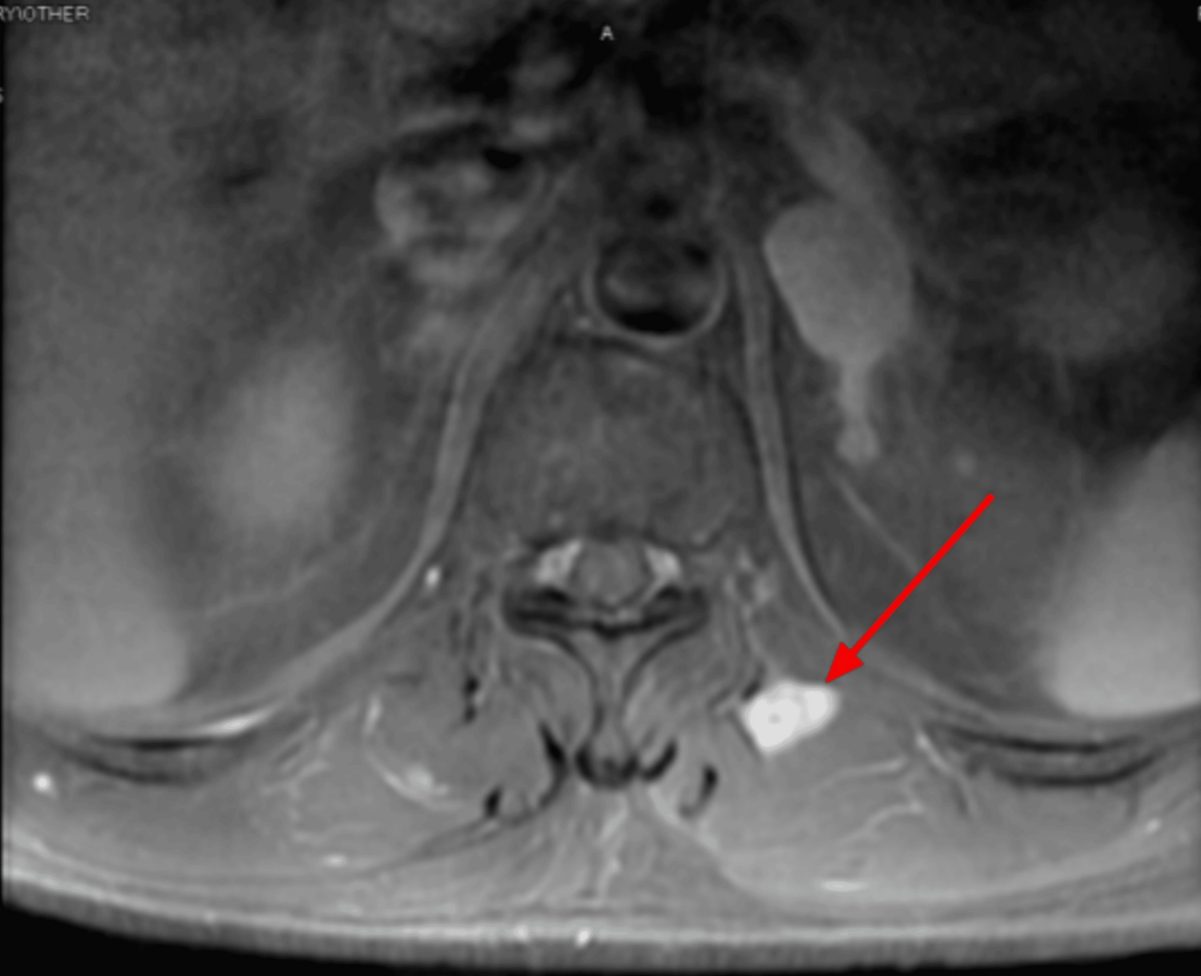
Chest CT (April 12, 2023) showing an enhancing hemorrhagic lesion in the soft tissue
